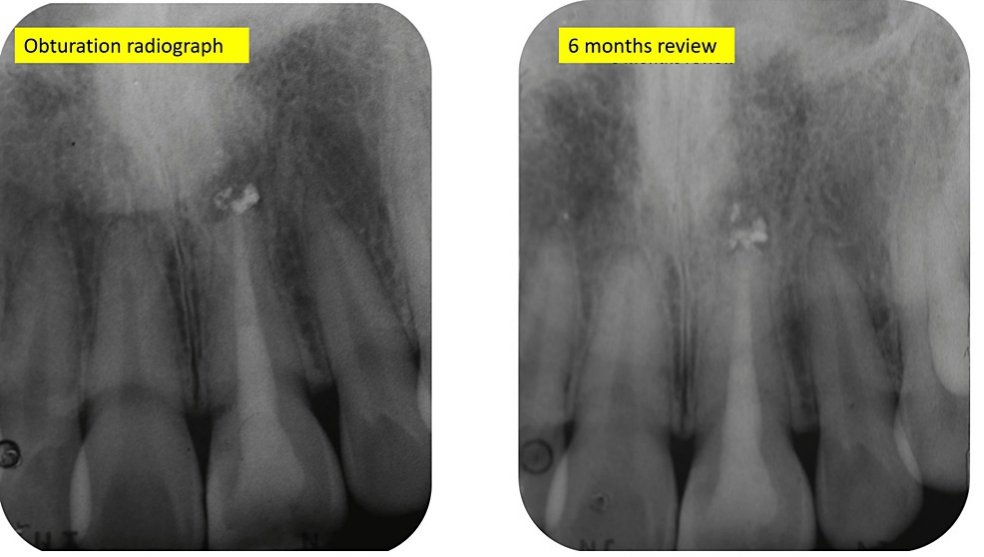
Postoperative radiograph and six-month review radiograph of tooth 21
